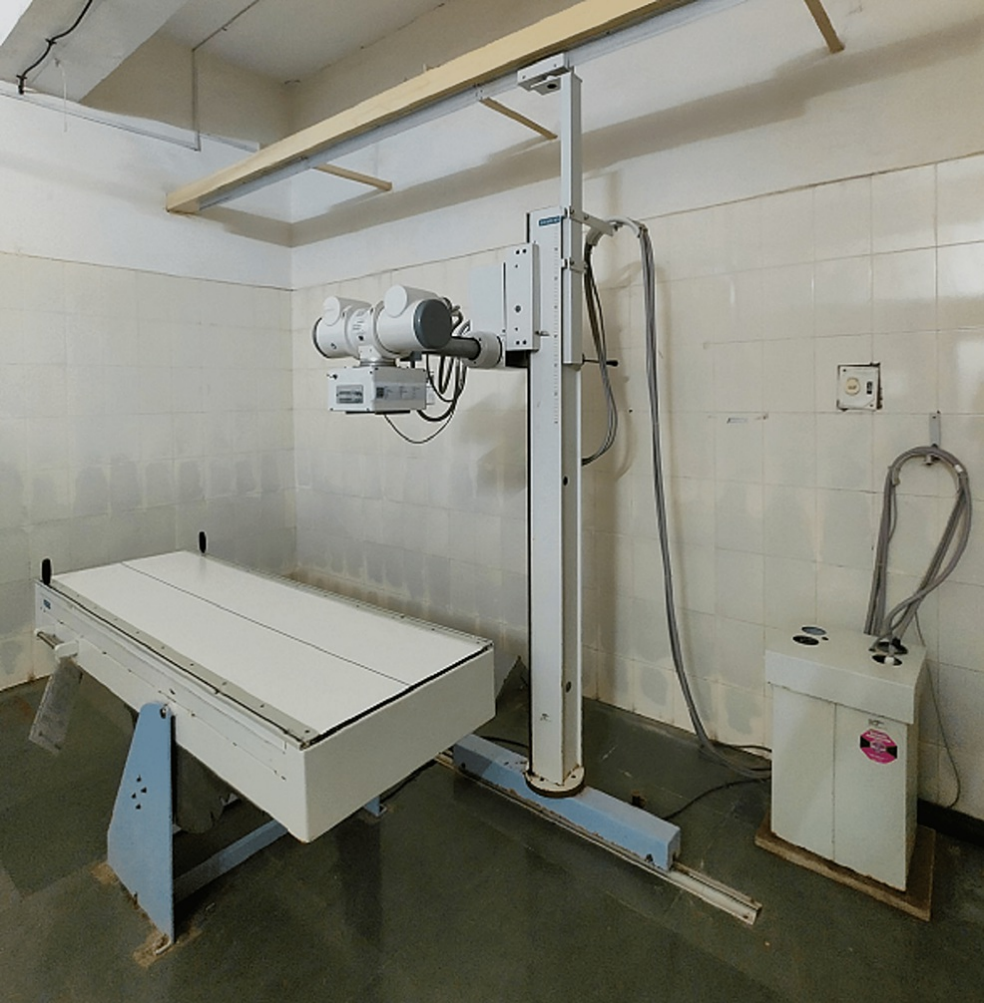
X-ray machine Image credit: Harsh Lahoti
